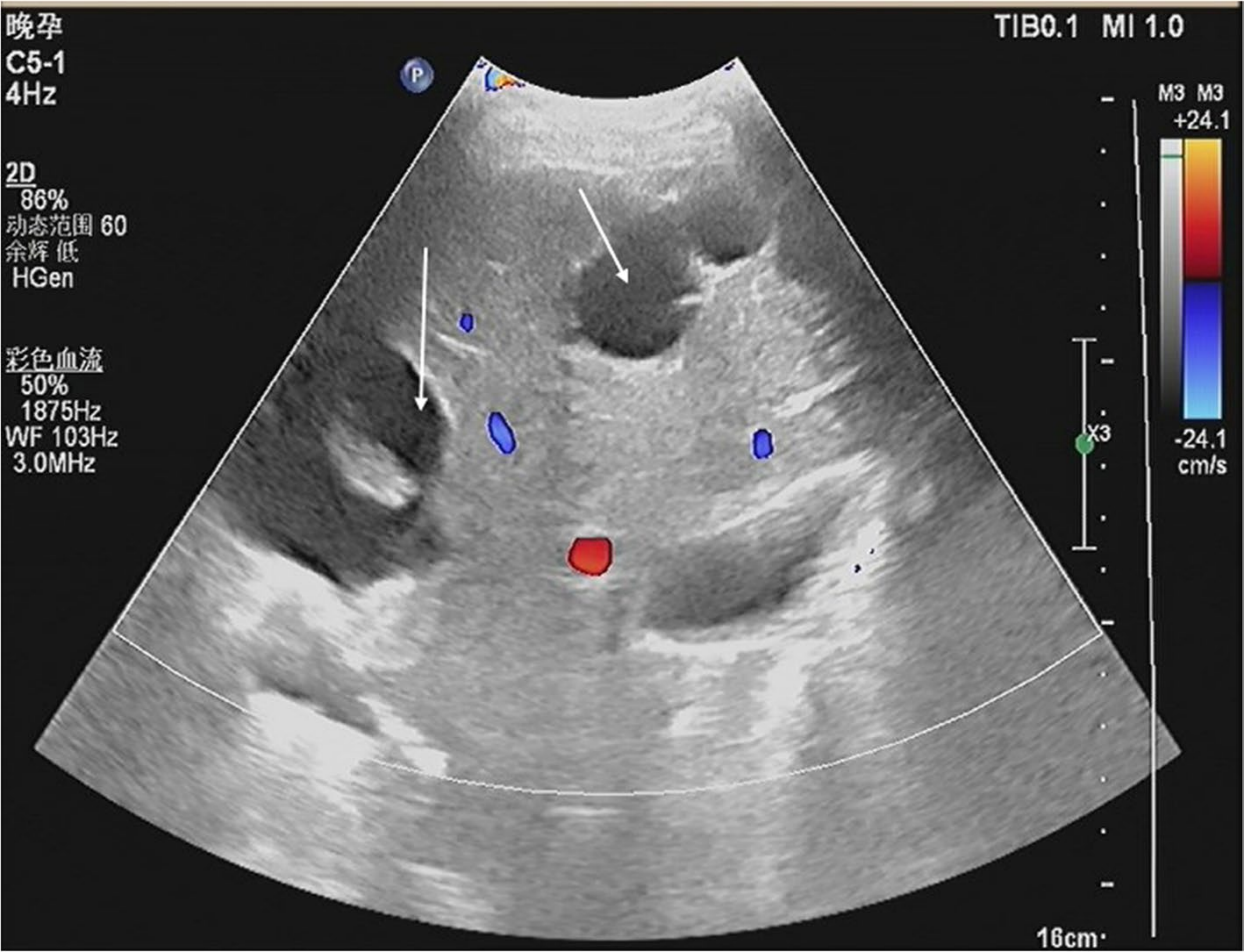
Sonography at 37 weeks of gestation. Multiple hepatic hydatids are shown (the largest cyst: 6.0*4.3 cm) (arrow)
